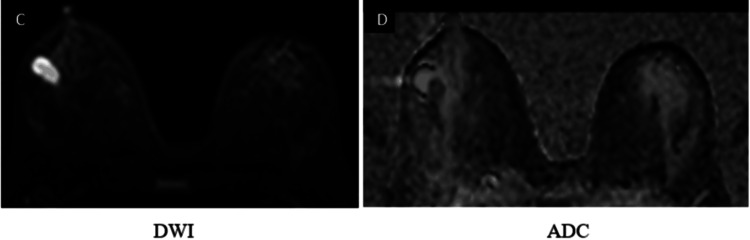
DWI and ADC maps (C, D) demonstrate marked diffusion restriction in the solid component of the lesion DWI: diffusion-weighted imaging; ADC: apparent diffusion
